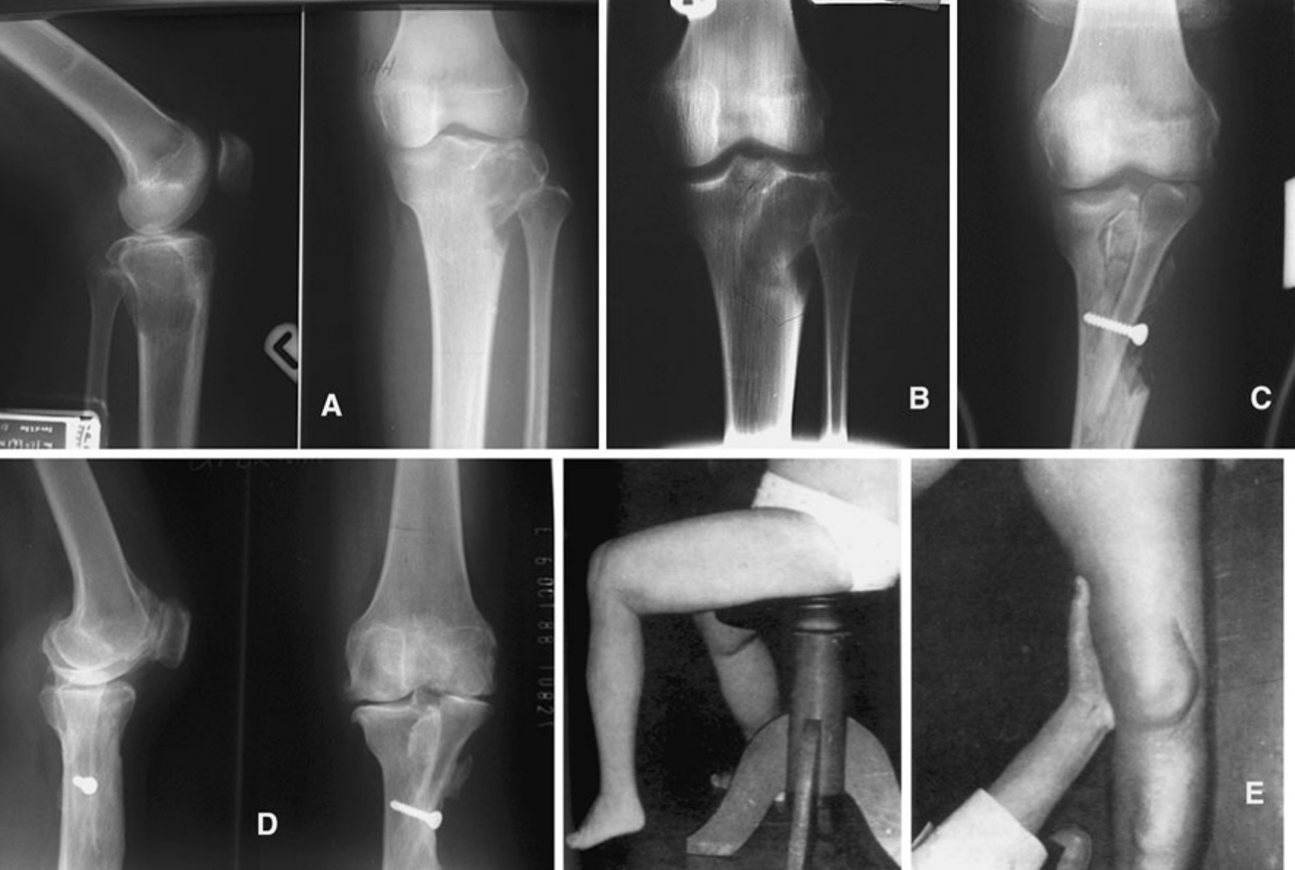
Early result. a Preoperative X-ray showing aggressive giant cell tumour of the lateral tibial condyle. b AP tomogram showing the extent of lesion and possible joint penetration. c Early healing at 12 weeks, patient still in brace. d X rays at 5 years showing well preserved joint surfaces despite early arthritic change. e Early clinical photograph at 2 years
